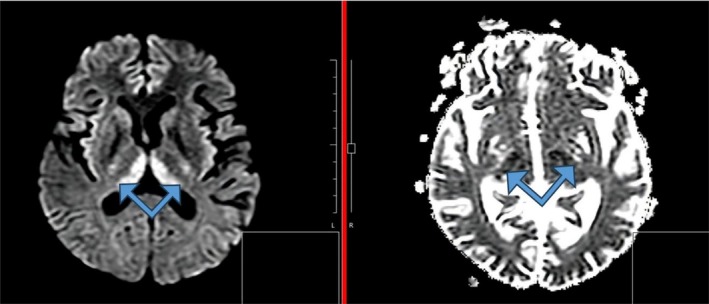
MRI of the brain showing diffusion restriction in the thalami.
